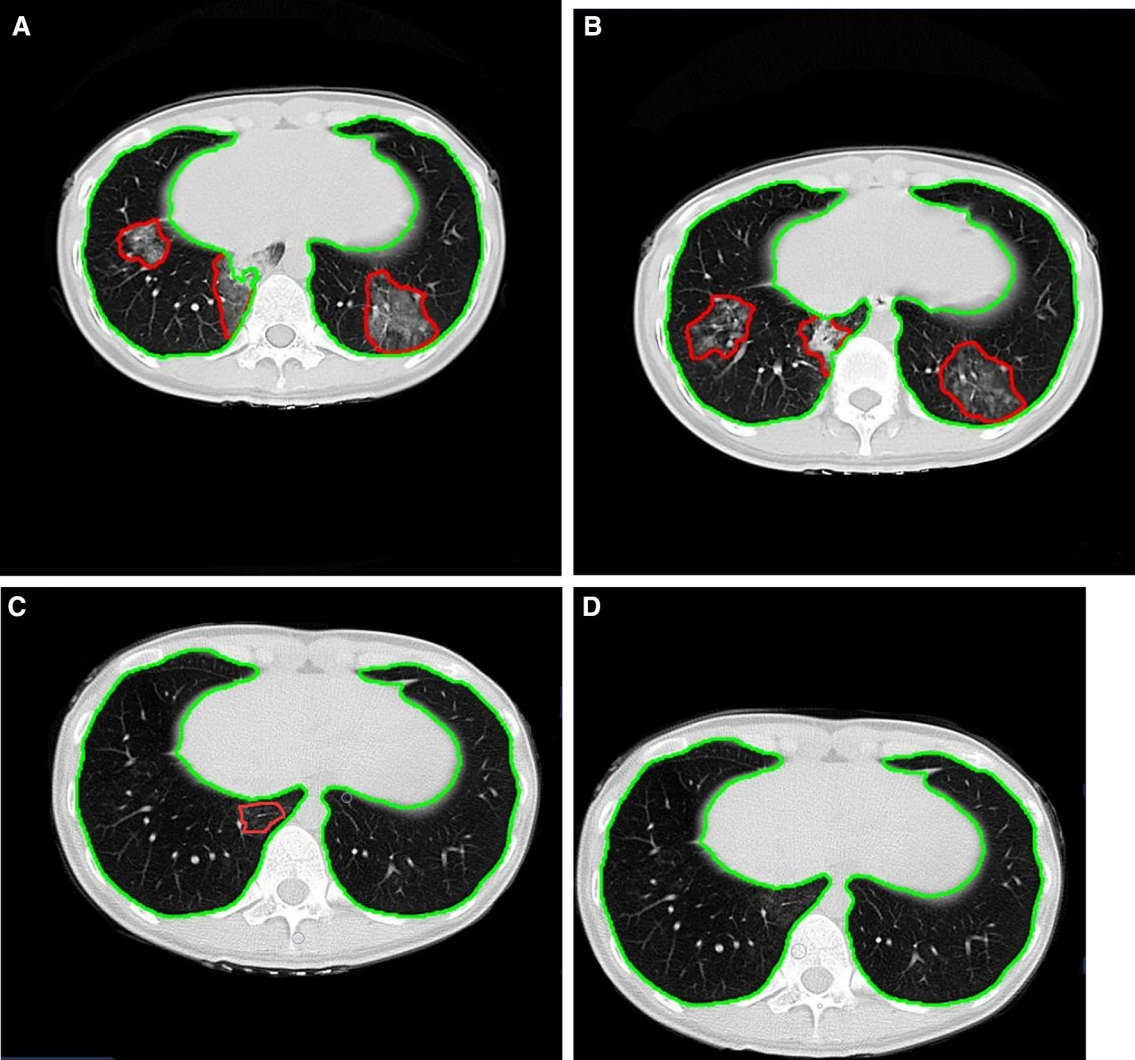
Longitudinal CT in a 24-year-old female hotel attendant exposed to COVID-19 briefly, but confirmed as MP. A Multiple GGO and consolidation shadows were observed in the lower lobes of both lungs at the baseline examination. B At the first follow-up, the volume of lung lesions was not progressed. C, D Lung lesion volume gradually decreased and then disappeared during the second and third follow-ups
